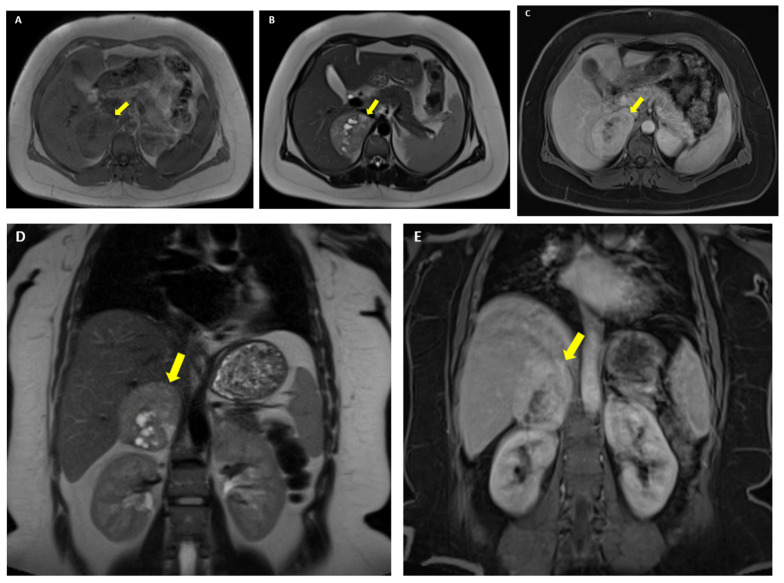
This is a 20-year-old adult who presented an episode of non-specific abdominal pain, initially regarded as a non-lithiasic cholecystic crisis. After abdominal ultrasound, further imaging evaluation was performed under these circumstances and led to the identification of a large right adrenal tumor at magnetic resonance imaging (MRI) [(A–E). Right adrenal tumor (yellow arrow) (A). MRI scan native phase, T1 WI FSE, axial plane: tumor of 7.2 by 5.3 by 6.9 cm, well-shaped, hypointense, without signal drop out on opposed phase sequence, with multiple cystic areas of maximum diameter 1.7 cm (the tumor compresses the medial margin of the right hepatic lobe and the inferior vena cava, while being well-separated from these structures); (B). MRI scan native phase, T2 WI HASTE, axial plane: tumor with intermediate/hyperintense signal, without signal drop out on opposed phase sequence; (C). Contrast enhanced MRI scan, T1 WI VIBE Dixon water-only, axial plane: tumor with hypointense signal, without signal drop out on opposed phase sequence, with moderate and slowly progressing gadolinium enhancement; multiple cysts insight the solid mass; (D). MRI scan native phase, T2 WI HASTE, coronal plane; (E). contrast enhanced MRI scan, T1 WI VIBE Dixon water-only, coronal plane].
